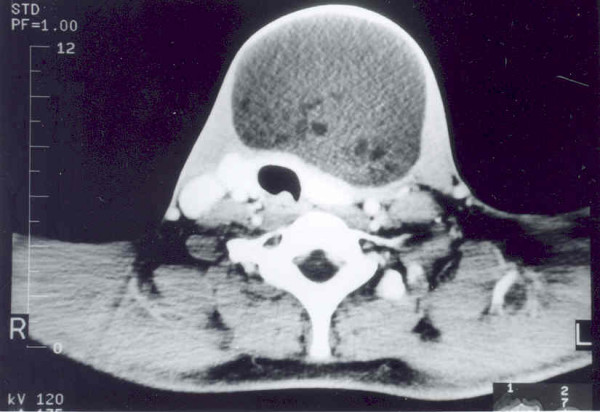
Contrast-enhanced CT scan of the neck showing a multi-septate cystic neck mass, lying superficial to the thyroid lobes and strap muscles.
